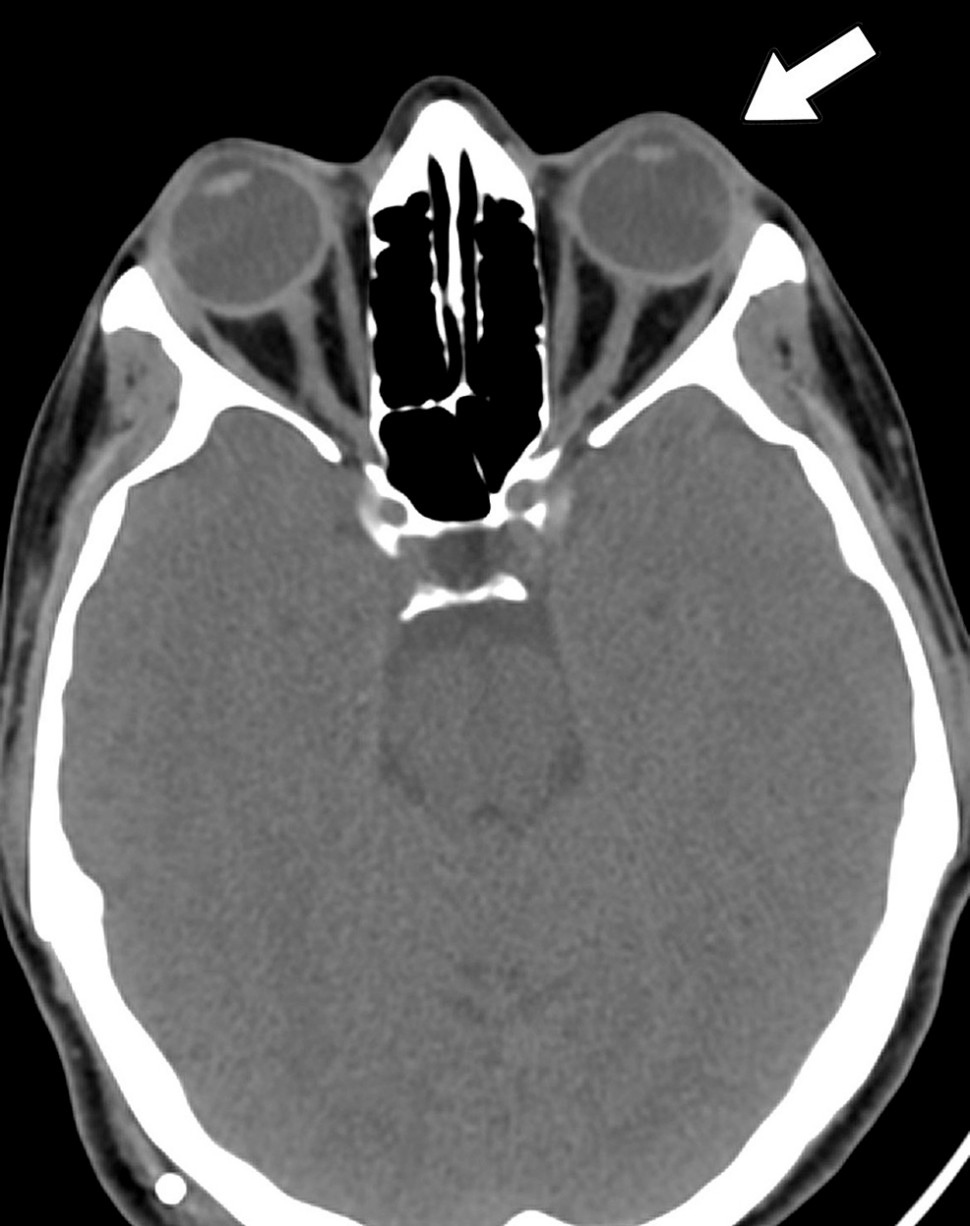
Postoperative CT axial view showing interval normalization (white arrow) CT: computed tomography
